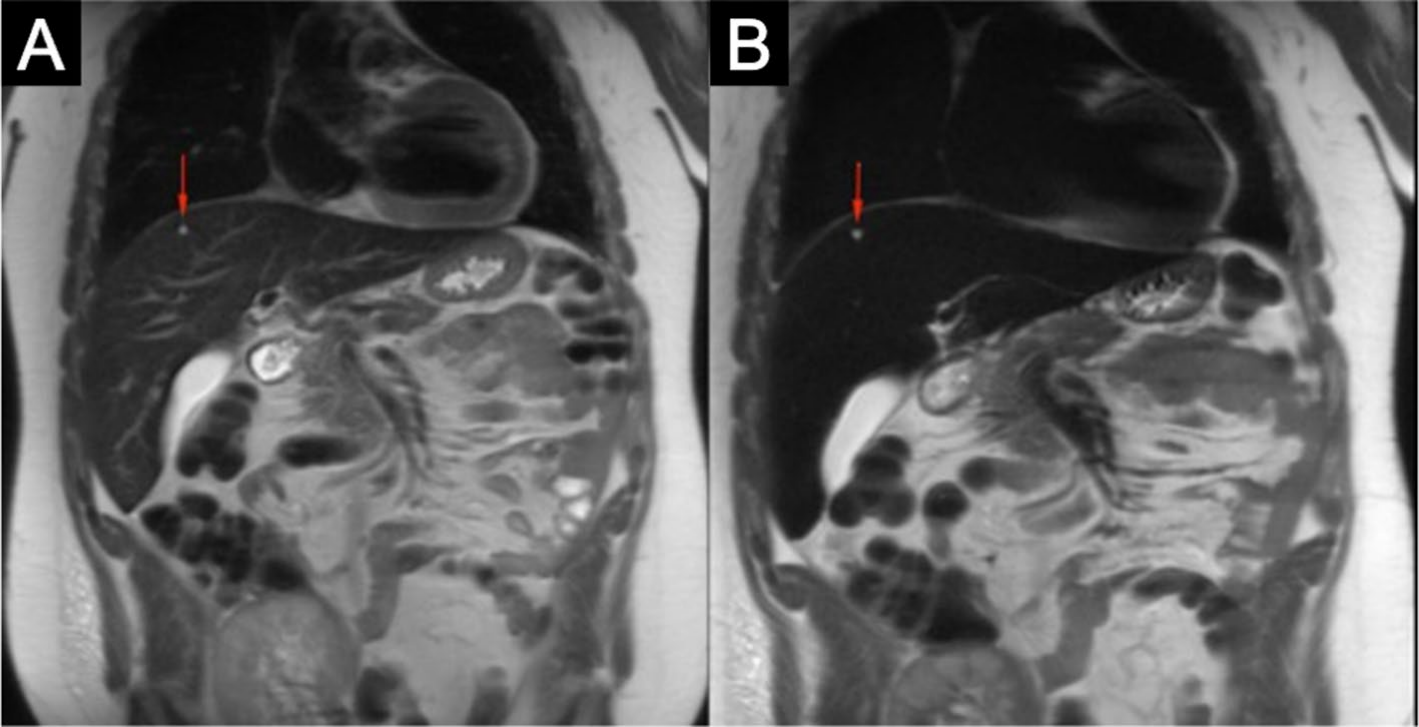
3.0 T ferumoxytol-enhanced MRI in a 20 year-old male with a single hepatic cyst. Pre- (a) and post-contrast (b) T2 acquisitions show increased conspicuity of a 4 mm hyperintense benign cyst (red arrow) as the normal hepatic tissue's signal intensity is suppressed
